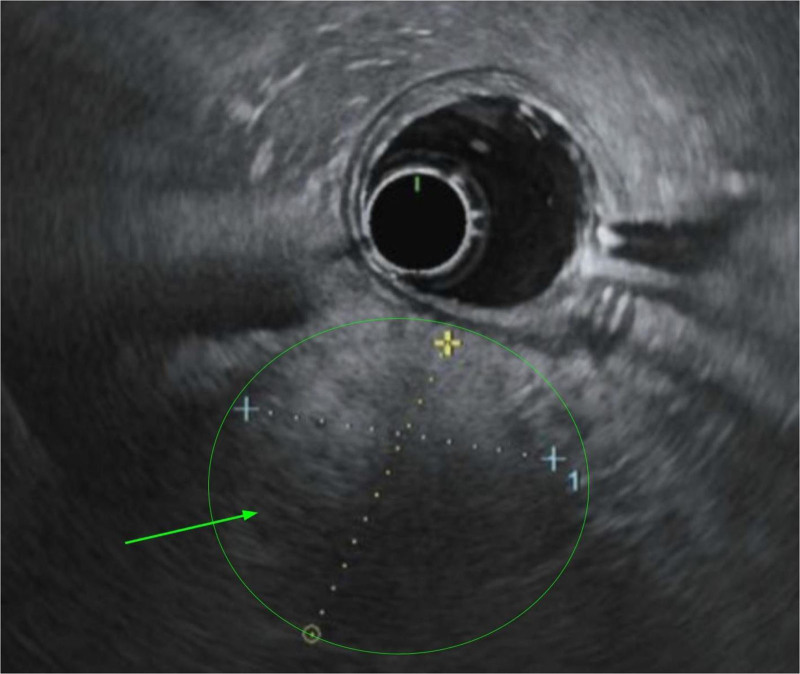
Endoscopic ultrasound of the pancreatic mass. EUS showed 3.4 cm heterogenous mass-like area in the pancreatic head (green arrow) concerning autoimmune changes versus malignancy. The mass-like area was subsequently biopsied. EUS = endoscopic ultrasound.
